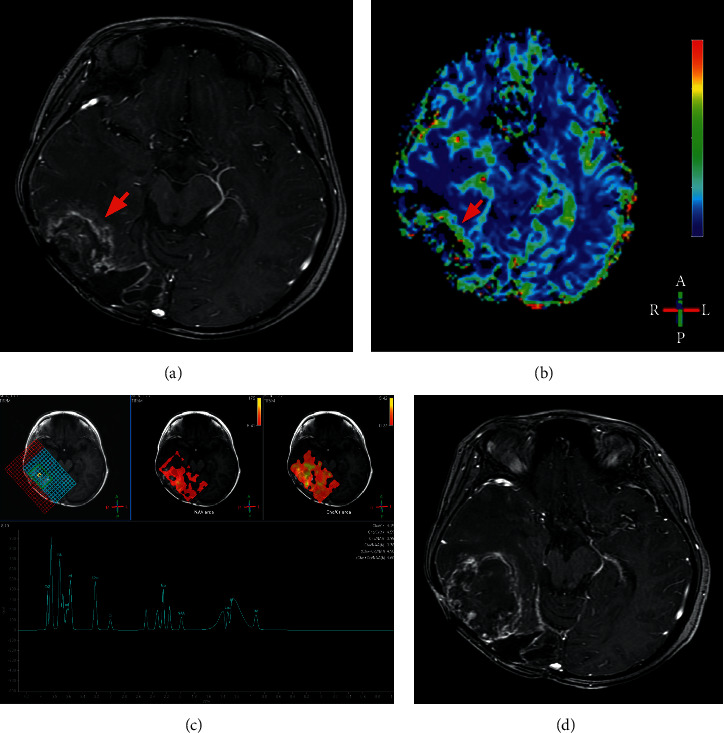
Example of true progression. An 18-year-old woman with pathologically proven glioblastoma. A new irregular ring enhancing lesion (arrow) developed in her right temporal-occipital lobe three months after completion of radiotherapy (a). Hyper-perfusion (arrow) was detected on her DSC-MRI (b), with rCBV value of 1.11. The MRS display Cho/Cr ratio of 4.15 and Cho/NAA 3.95 (c). The enhancing lesions enlarged significantly one month later (d). Tumor recurrence was confirmed pathologically after reoperation.
